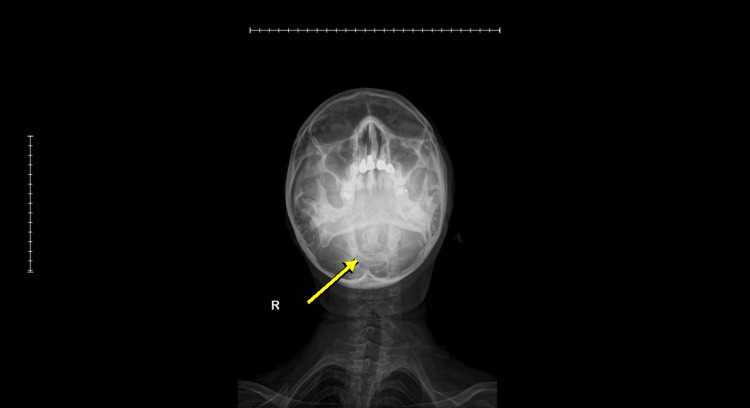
Base posterior (vertex) radiograph. Arrow indicates the upper cervical joint region.
